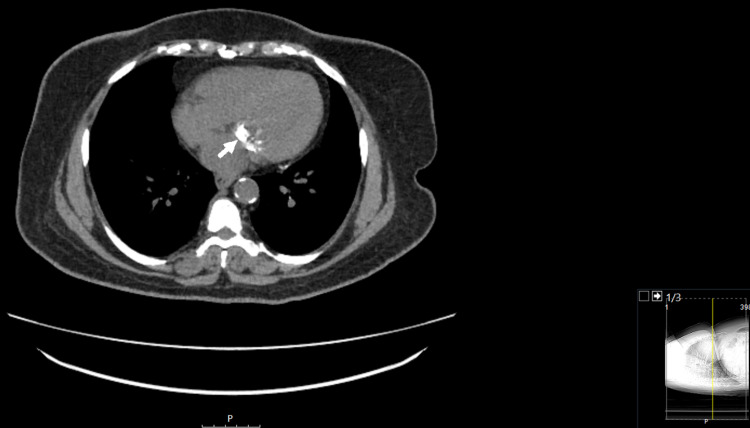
A computed tomographic chest scan view without contrast. 1 mm slices taken showing dense mitral valve annular calcification predominantly involving the inferior aspect of the annulus (white arrow). Calcifications in the aorta are also seen.
